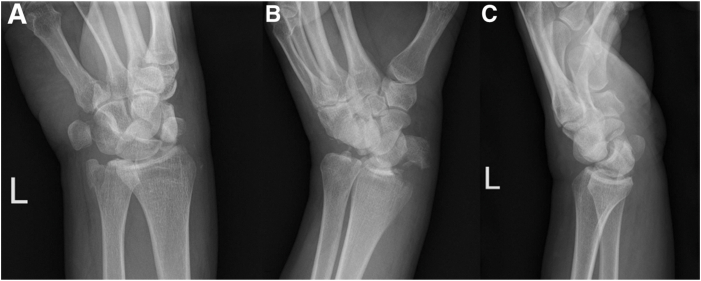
(A–C) Radiographic imaging of A 50-year-old left hand–dominant male, who sustained an injury to his left hand after a snowmobile accident. After assessment in the emergency department, x-ray imaging was ordered. PA, Oblique and lateral x-rays demonstrated a trans-radial, trans-ulnar styloid perilunate injury, with breaks in Gilula’s lines.
